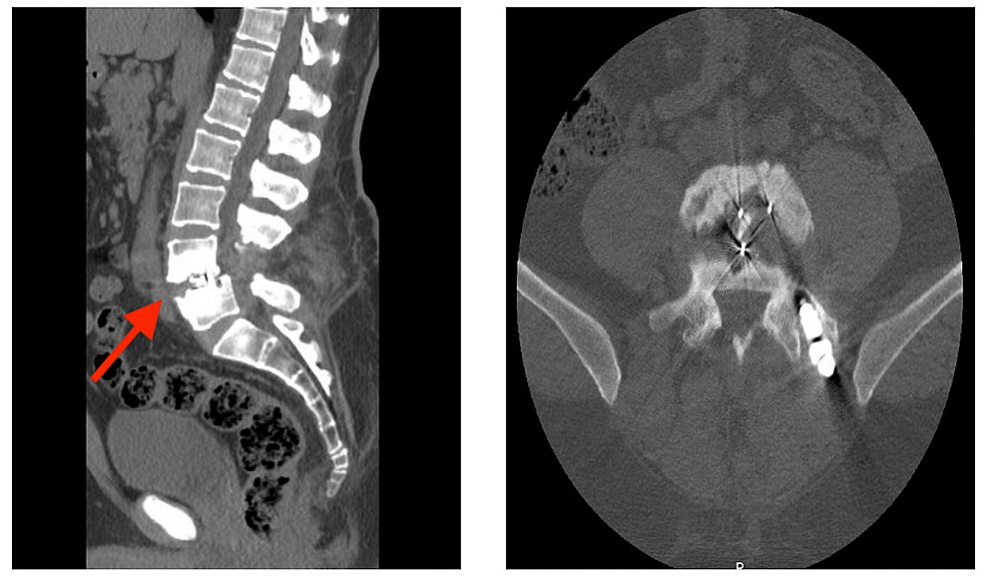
Pre-revision CT scan. CT scan reported as sclerotic L4-L5 opposed vertebral endplates (red arrow) with irregular outlines and no fusion can be appreciated.
